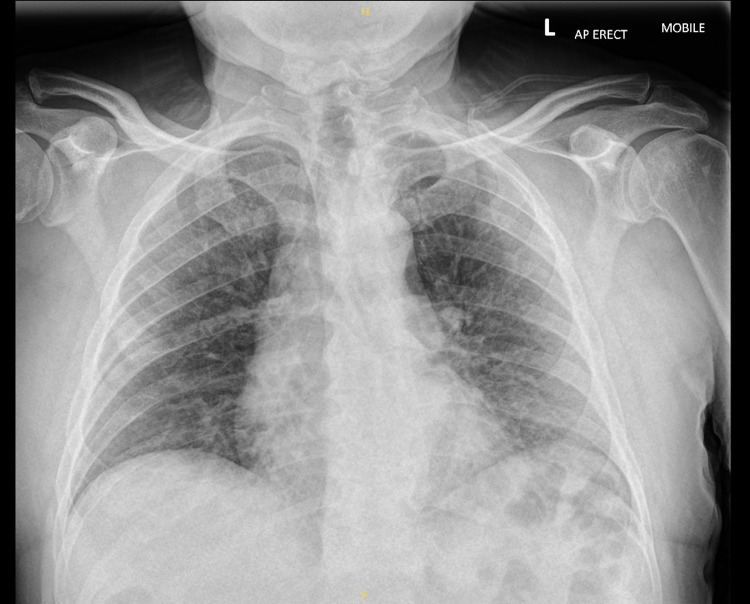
Admission chest X-ray demonstrating only mildly increased lung markings
